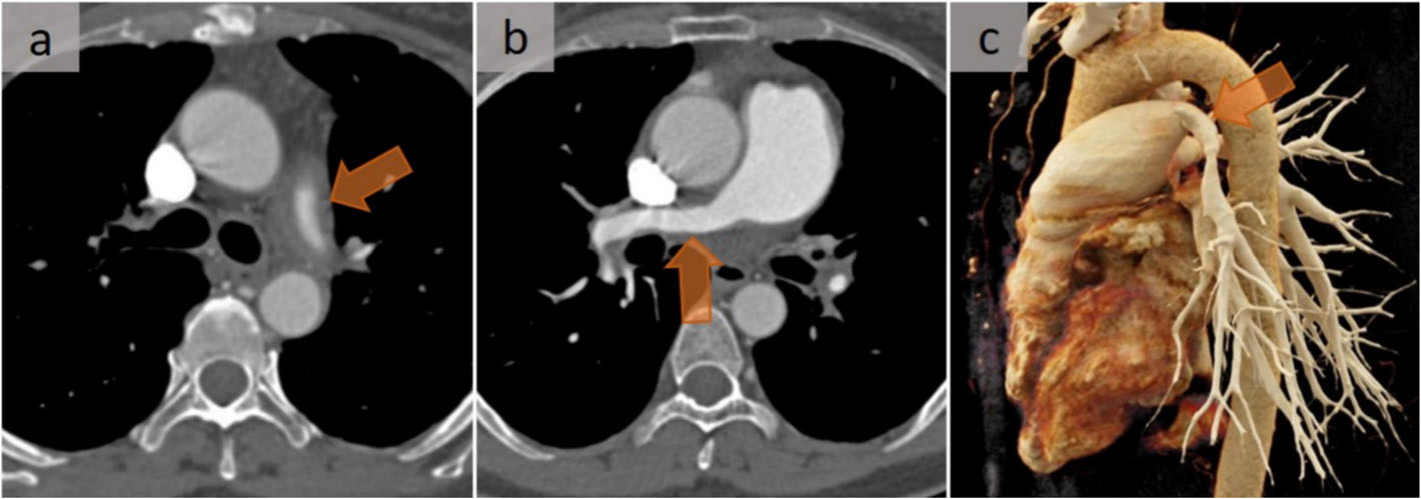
Post-mediastinitis pulmonary hypertension. Pulmonary artery stenosis in a case of fibrosing mediastinitis with pulmonary hypertension. Contrast-enhanced CT scan demonstrated at two successive levels (a, b), with a soft tissue attenuation mass diffusely infiltrating the mediastinum with encasement and narrowing of the pulmonary arteries (arrows). The stenosis of the right pulmonary artery is perfectly observed on the oblique 3D rendering view (c) (arrow). This mass had no significant metabolic uptake on 18F-FDG PET/CT (not shown). Note also the stenosed upper pulmonary veins
